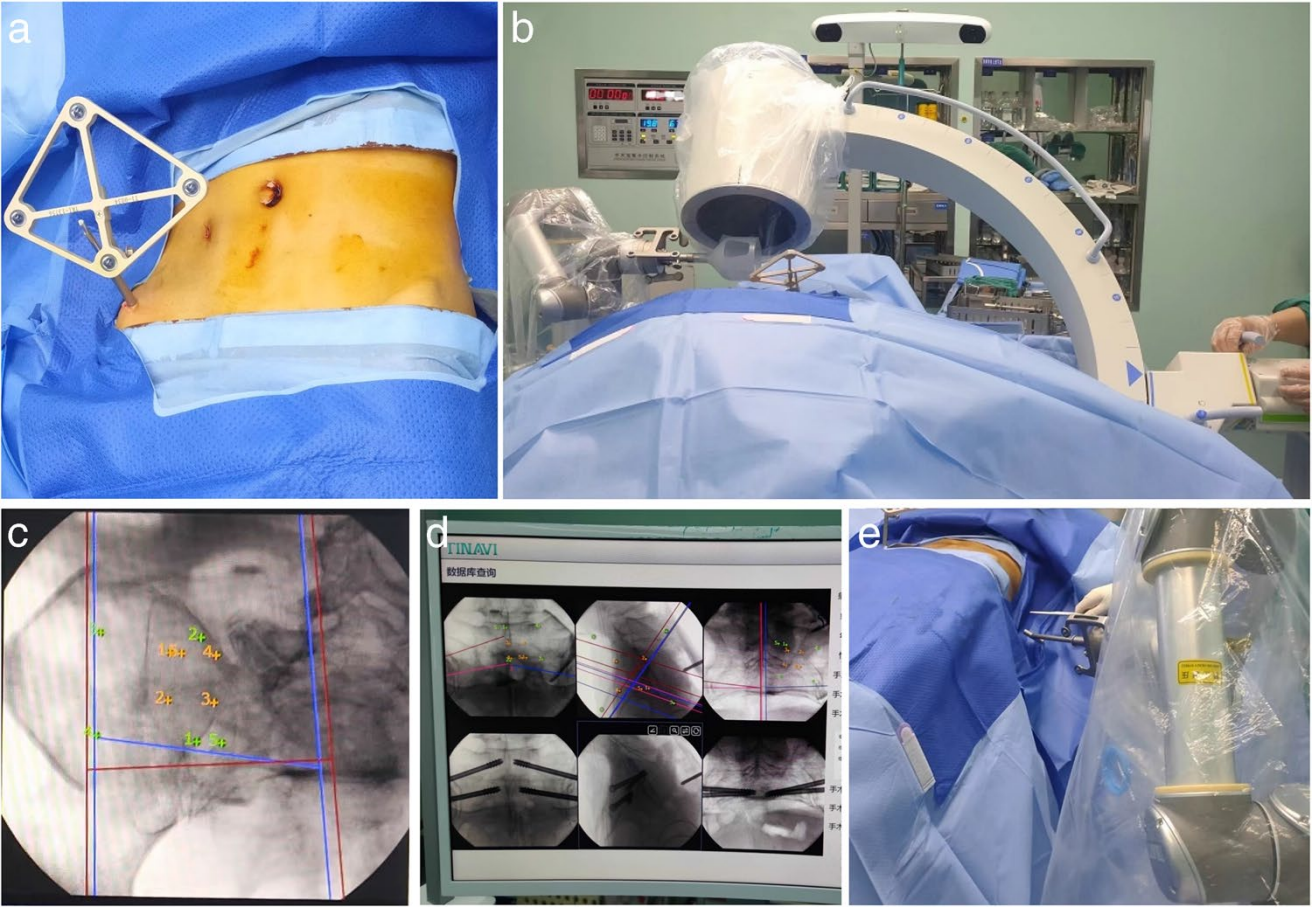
a A tracer is placed on the patient's anterior superior iliac spine by the surgeon. b Confirming all marks on the tool tracker by X-ray. c The entry point, angle, and length of screw were designed and locate the screw’s entry point and direction. d The computer plans the position of the screw. e The operator inserts the guide wire into the guide sleeve
